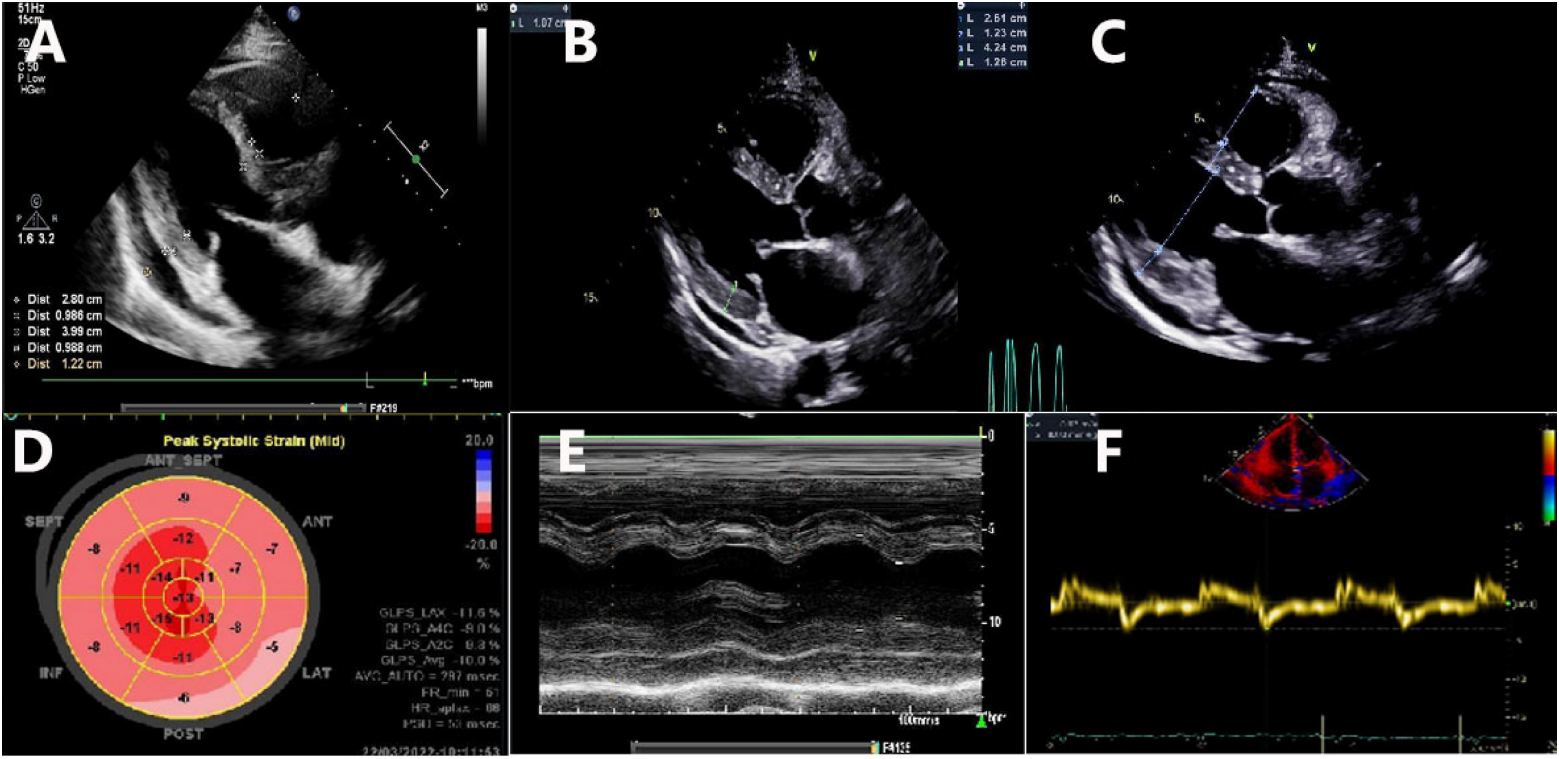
Transthoracic echocardiographic imaging. (A) A long axis view shows the normal wall thickness of the IVS (9.86 mm) and LVPW (9.88 mm) obtained on 6 March 2022; (B) a long axis view shows the normal wall thickness of the IVS (10.5 mm) and LVPW (10.7 mm) obtained on 22 March 2022; (C) a long axis view shows the increased wall thickness of the IVS (12.3 mm) and LVPW (12.6 mm) obtained on 21 May 2022; (D) decreased global longitudinal strain (GLS − 10%) and a pattern of relative “apical sparing” of longitudinal strain in the apex of the LV; (E) normal left ventricular wall motion; (F) reduced tissue Doppler mitral annular velocities at the septal mitral annulus.
